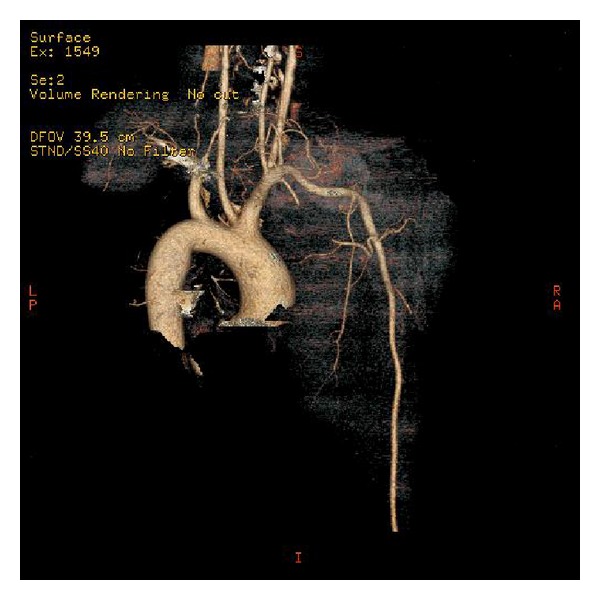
Preoperative CT-guided angiography displaying no vascular involvement but close proximity.
